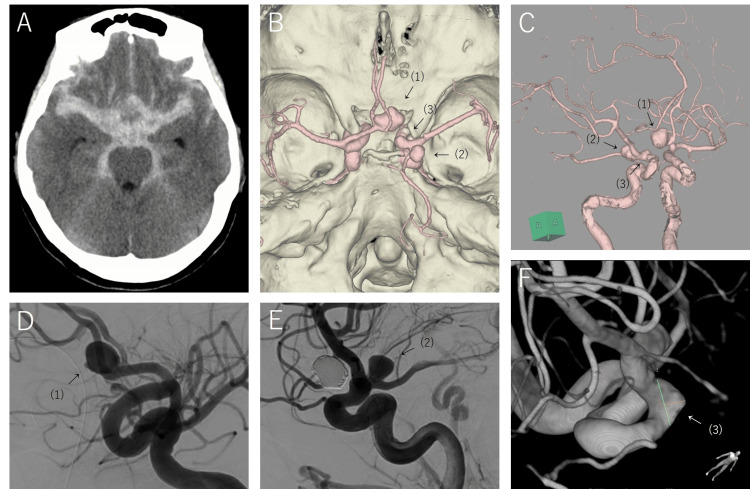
(A) Initial brain computed tomography revealed subarachnoid hemorrhage. (B, C) Computed tomography angiography showed an anterior communicating artery aneurysm (1), a true posterior communicating artery aneurysm (2), and an anterior wall aneurysm of the internal carotid artery (3). (D) Preoperative angiography of the anterior communicating artery aneurysm. (E) Preoperative angiography of the true posterior communicating artery aneurysm. (F) Preoperative angiography of the anterior wall aneurysm of the internal carotid artery.
